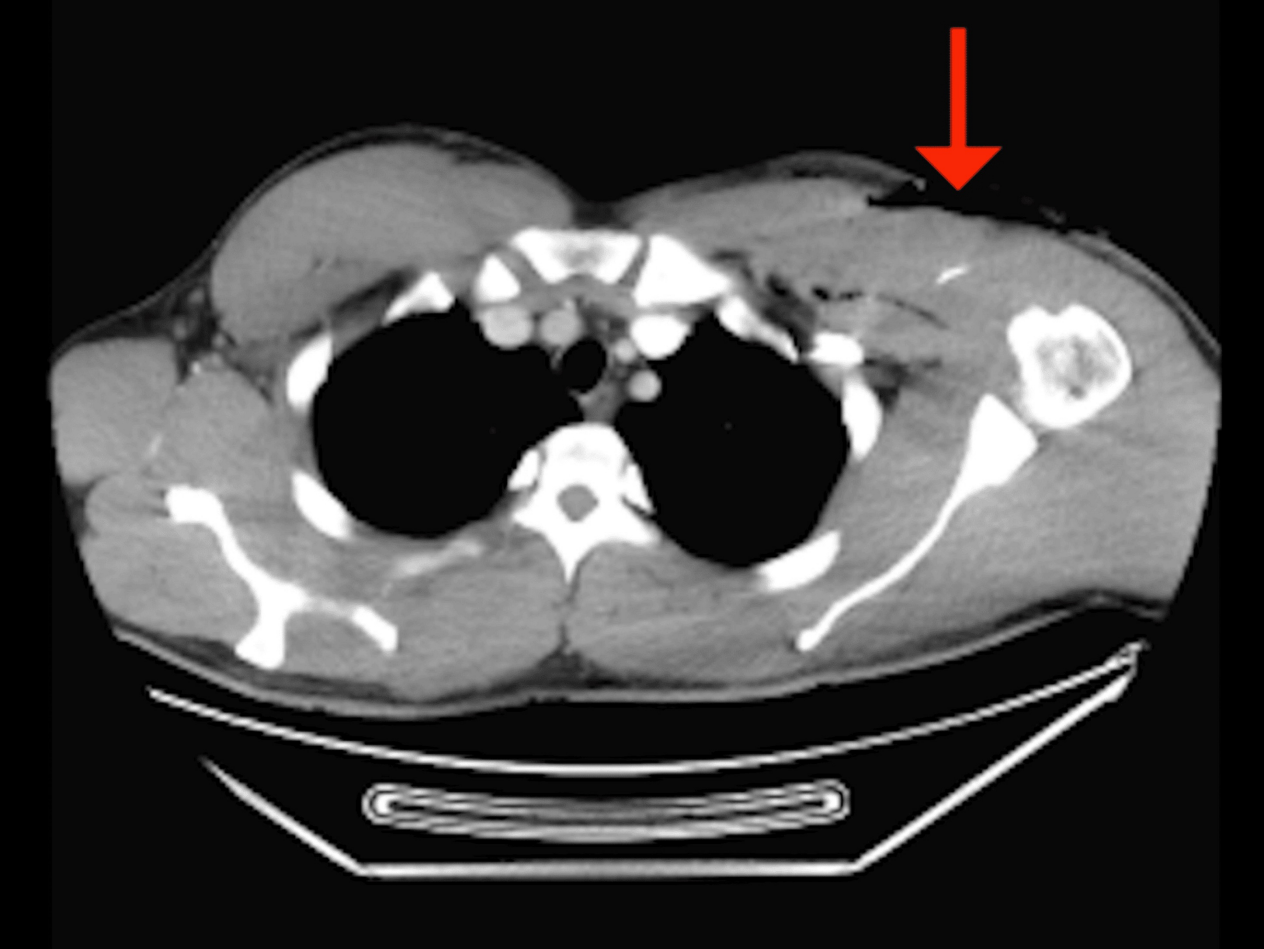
Chest CT showed soft tissue disruption on the left anterior upper chest wall, accompanied by a significant skin defect, muscle loss, and subcutaneous emphysema surrounding the left pectoralis major.
